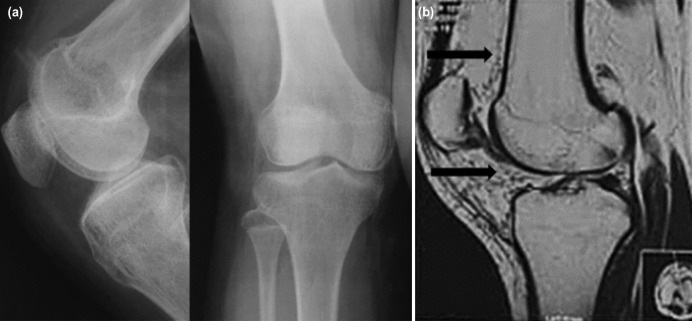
(a) Plain radiograph of the right knee at initial presentation showing no significant bony abnormality or soft tissue swelling. (b) The MRI of the right knee suggests infection, . reported as an aggressive lesion, possibly osteomyelitis of the distal femur with subperiosteal abscess associated with enhancing synovium (black arrow). The mixture of high and low signals within the synovium might represent highly vascularised synovial tissues with low signals hemosiderin deposited tissues.
